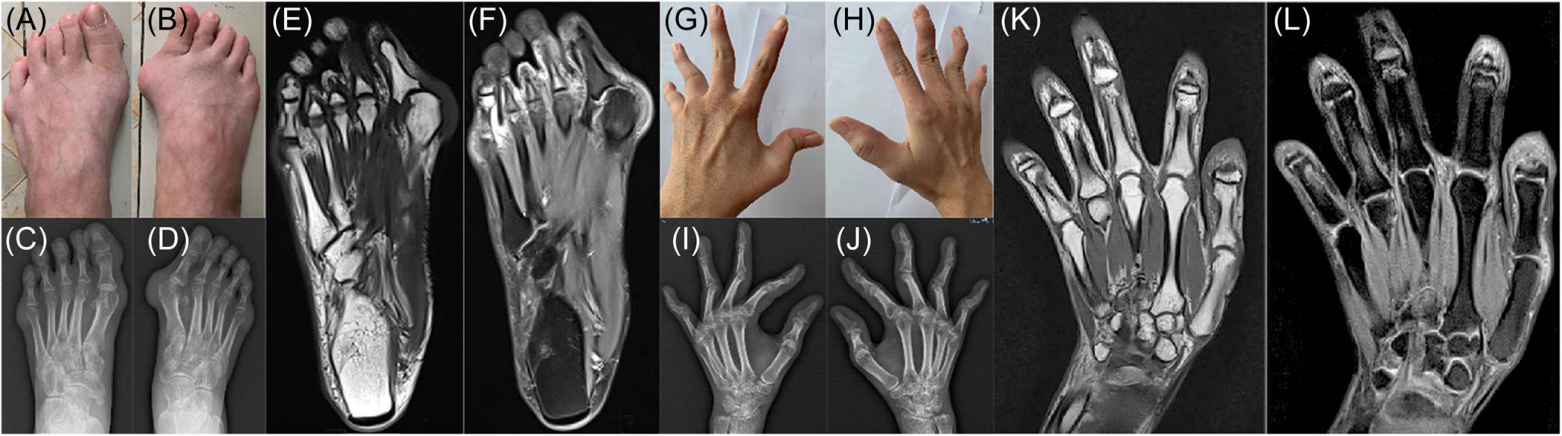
Jaccoud's arthropathy. (A, B) Clinical photographs of both feet with swollen joints and hallux valgus. (C, D) Radiography of both feet with luxation and subluxation of the joints. (E, F) The magnetic resonance image (MRI) of the right foot with synovitis. (G, H) Clinical photographs of both hands with swollen joints, swan neck deformities, and ulnar deviation. (I, J) Radiography of both hands with luxation and subluxation of the joints. (K, L): the MRI of the right hand with synovitis.
